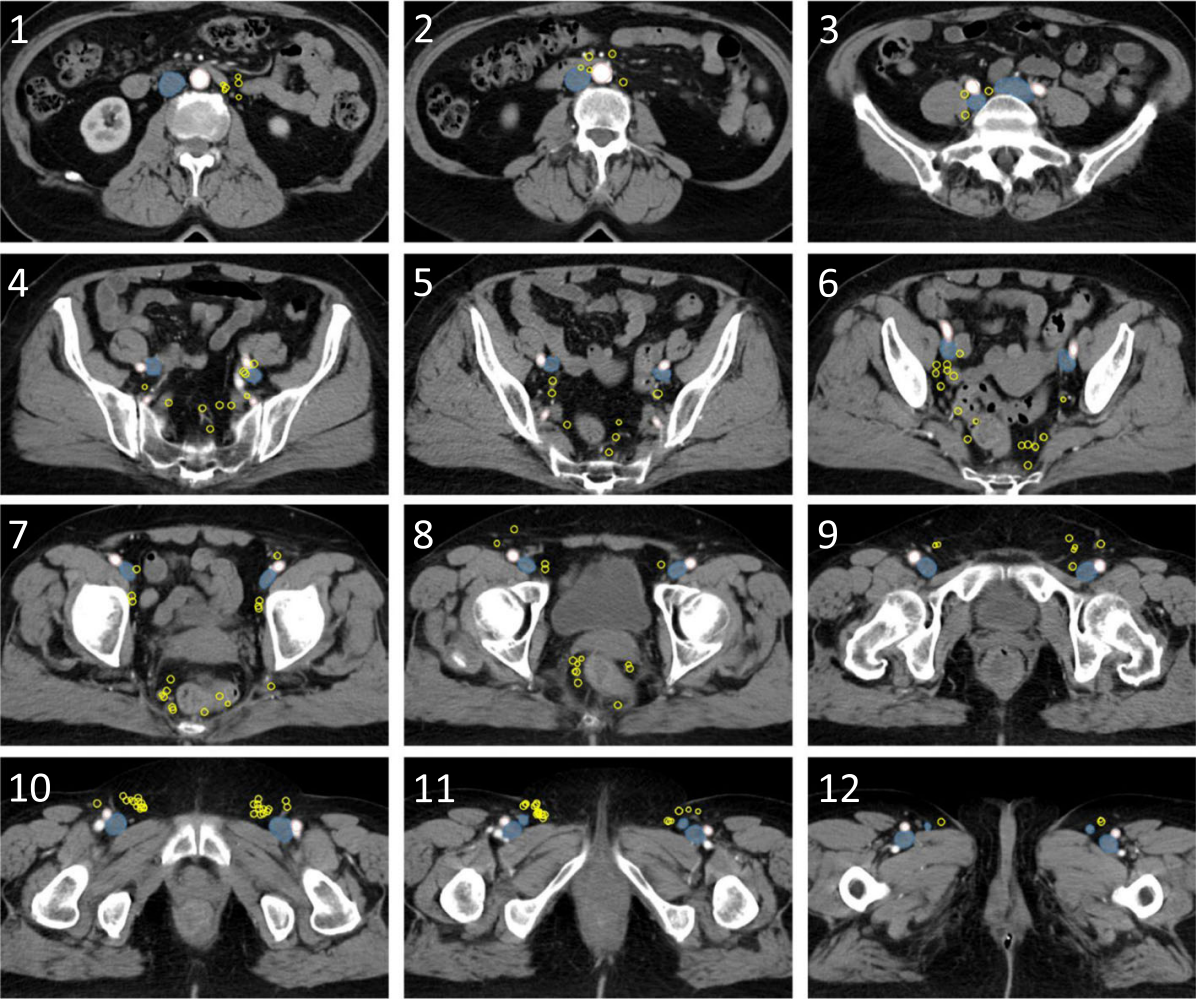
Axial CT slices with PET-positive lymph nodes (*yellow*) in all patients. Center of lymph node mapped with a 6 mm sphere in a standard anatomy reference CT. *Red*, arteries. *Blue*, veins
